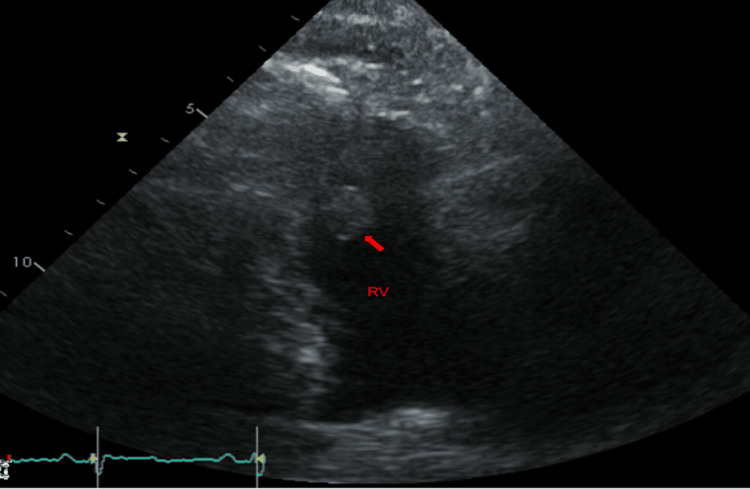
2D echocardiogram. Right ventricular (RV) cavity with a large mass.
